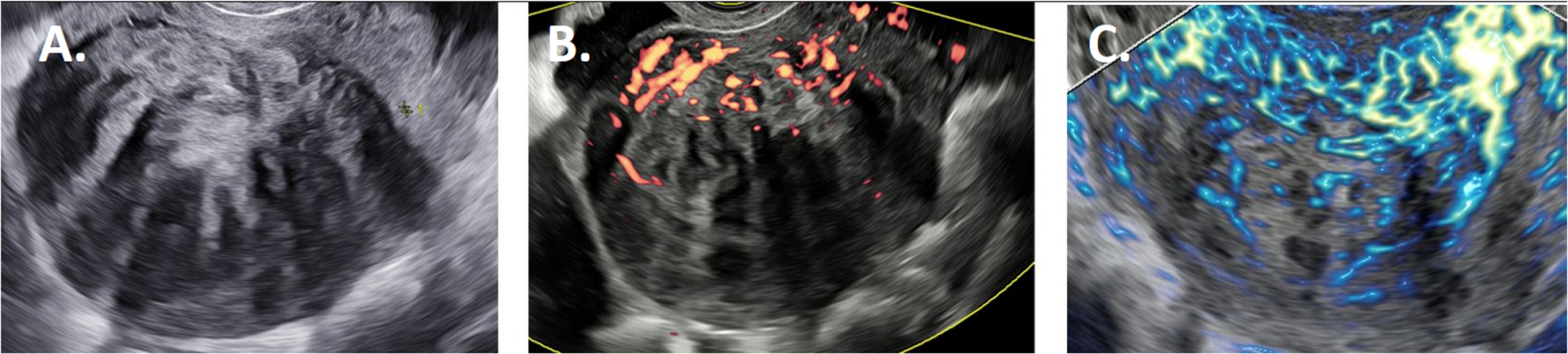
Sagittal fibroid images obtained by the different ultrasound techniques in patient 1. 2D B-mode ultrasound (A); 2D Power Doppler (B); 2D Microvascular flow ultrasound (C)
